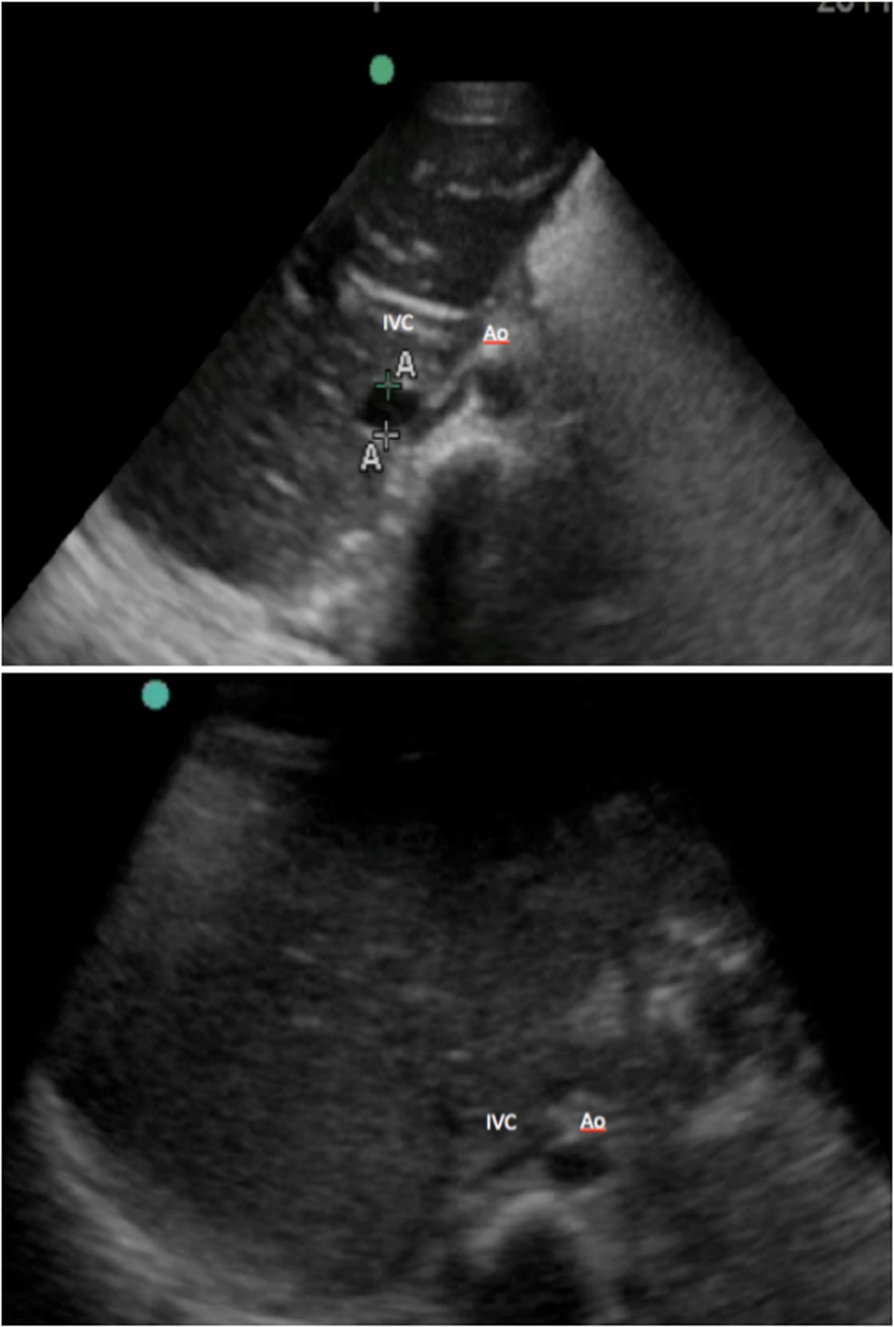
Ultrasound image of the transverse inferior vena cava to aorta (IVC/Ao) diameter measurement in a child with an IVC/Ao ratio >0.8 (no dehydration) above and <0.8 below (significant dehydration). Ao, aorta; IVC, inferior vena cava.
